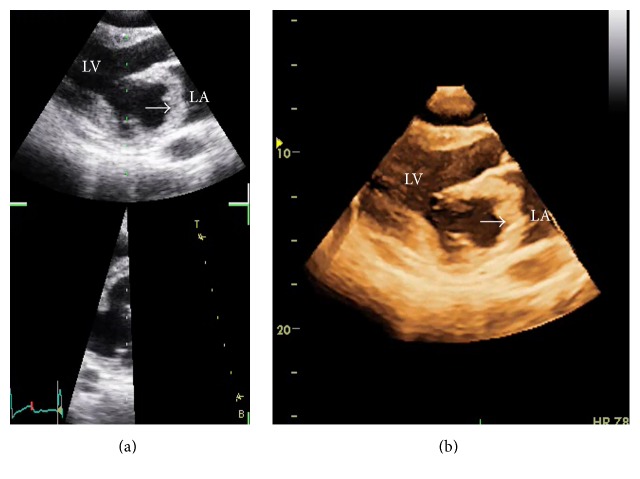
Transthoracic 2D (a) and 4D (b) echocardiographic views in parasternal long axis orientation. Parachute-shaped mass (arrows) in LA attached to atrial side of AML and inferoposterior region of left atrial endocardium. LA: left atrium; LV: left ventricle.
